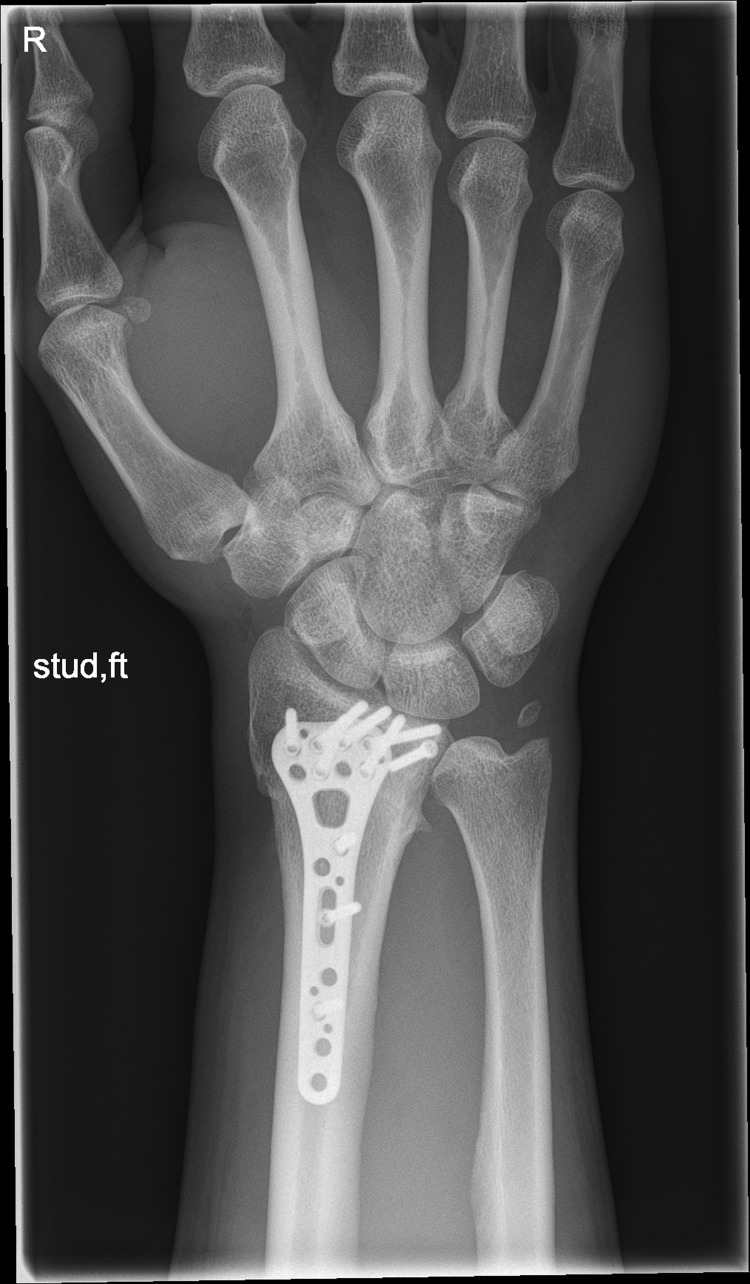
Dorsovolar and lateral X-ray control 1 year after surgery
